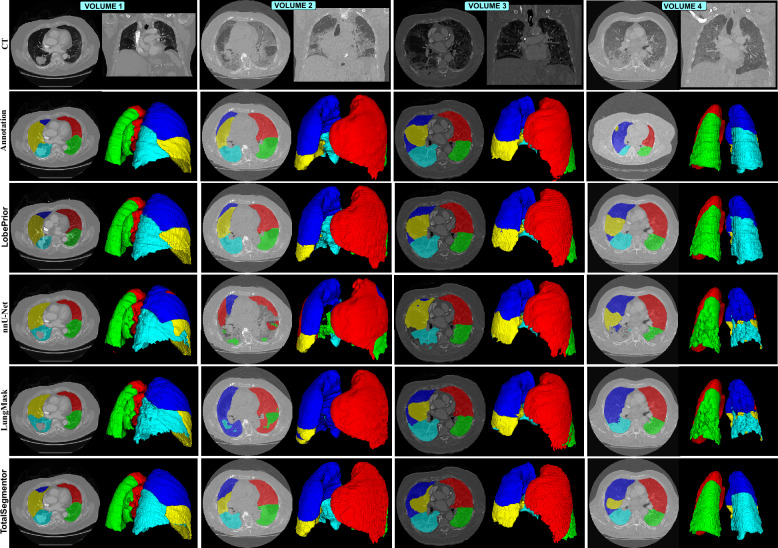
Qualitative results on CT images from the LOCCA dataset. Each column pair includes an axial view in the first column and a rendering of the lung lobes in the second, except for the first image in the second column, which is a CT image in the coronal view. The images in the second row correspond to manual annotations performed by the authors. The first two columns (volume 1) are from cancer patients, containing a moderate-type lesion. The remaining images are from COVID-19 patients and present severe-type lesions.
